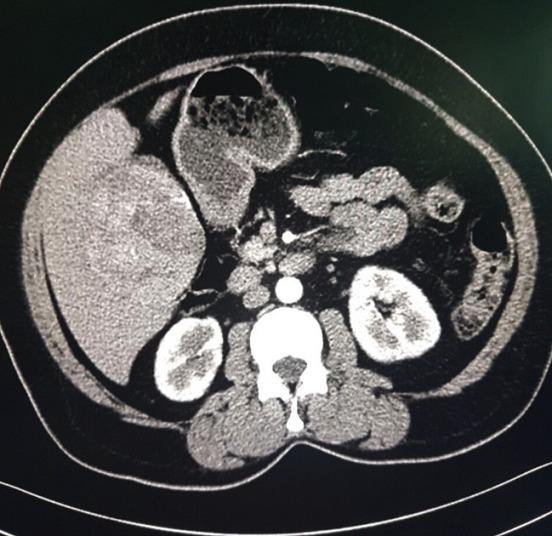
Abdominal CT scan showing a hepatic tissular mass that displays an arterial contrast enhancement
